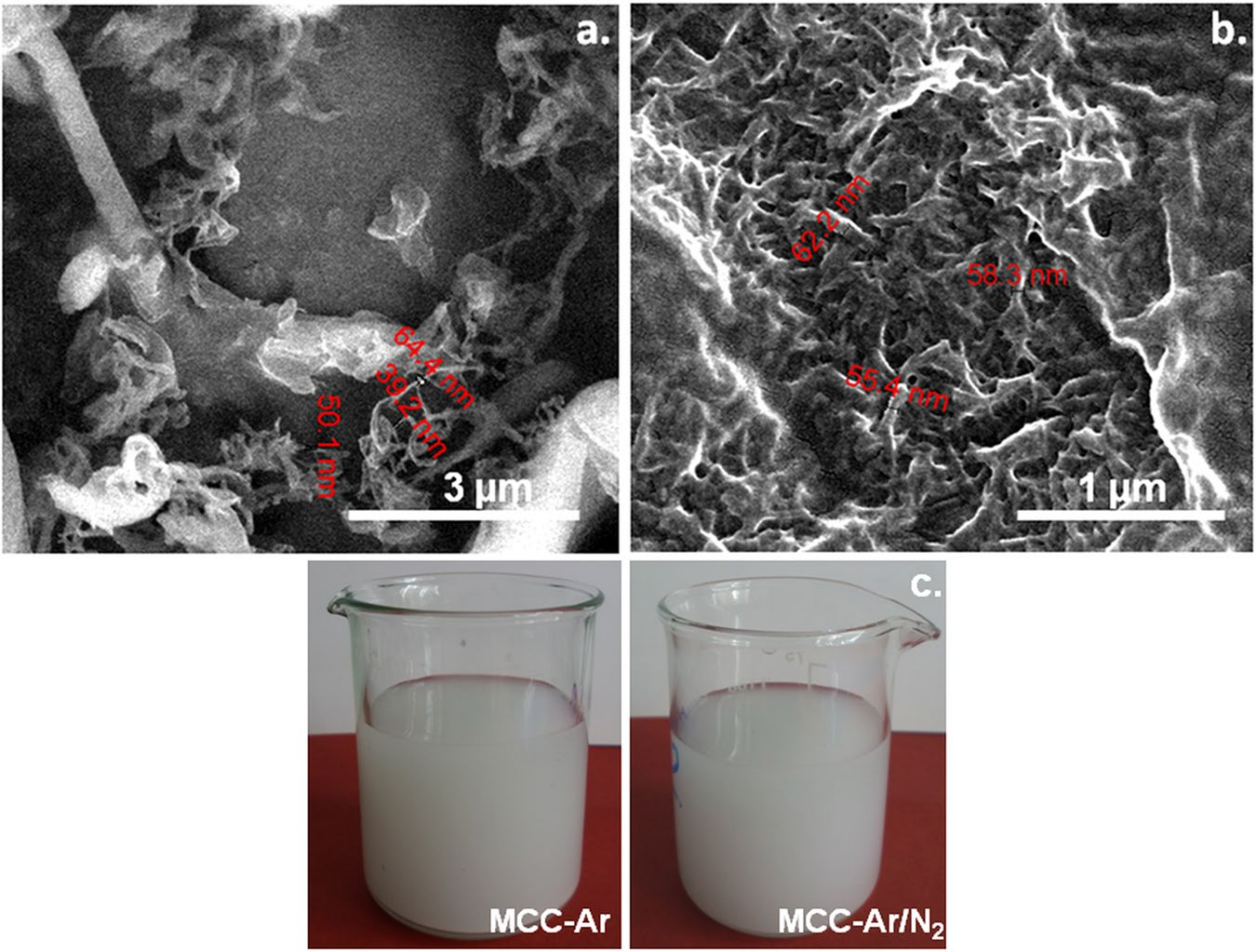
Detailed SEM images of Ar (a) and Ar/N_2_ (b) plasma treated MCC; (c) the supernantant is turbid for both Ar and Ar/N_2_ plasma treatments (24 hours after ultrasonication).
